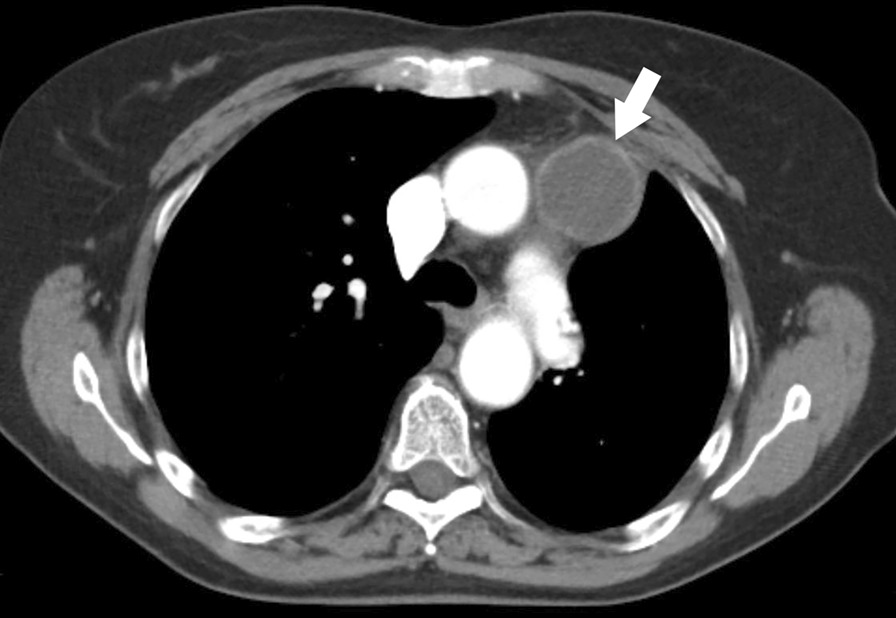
CT demonstrates metastatic disease involving a peripherally enhancing and centrally necrotic subaortic lymph node (arrow)
